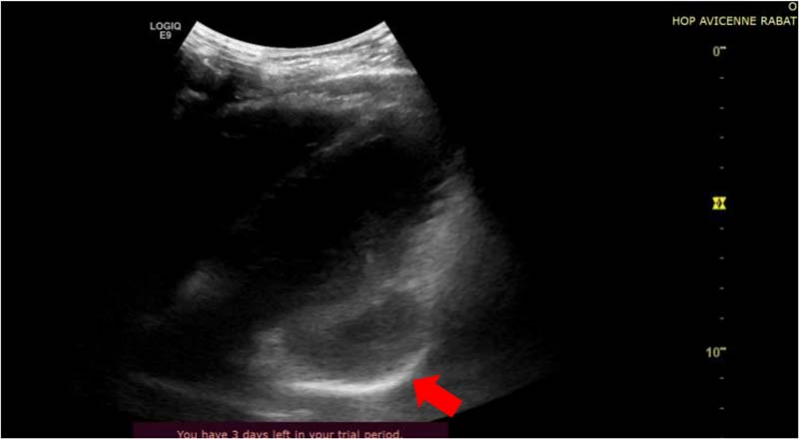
Trans-thoracic four chamber echocardiographic view showing a cystic mass (arrow) on the anterior lateral segment of the left ventricle.
